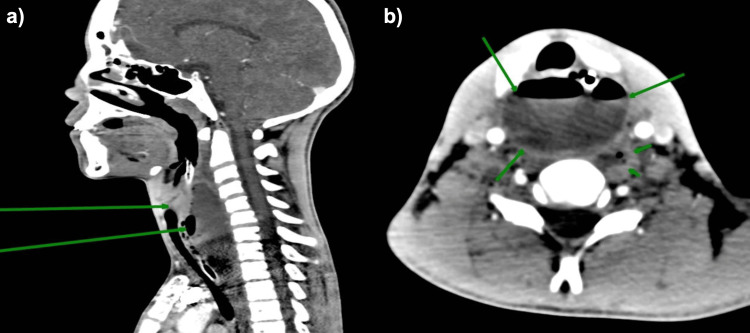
Neck CT angiography findings (a) Sagittal CT angiography view of the neck showing an extensive collection with pockets of gas and air-fluid level with enhancing walls in the retropharyngeal space extending from C3 to T3, displacing the trachea and compressing the esophagus. (b) Axial CT angiography view of the neck showing a larger abscess (long arrows) and a smaller abscess (short arrows). CT: computed tomography
